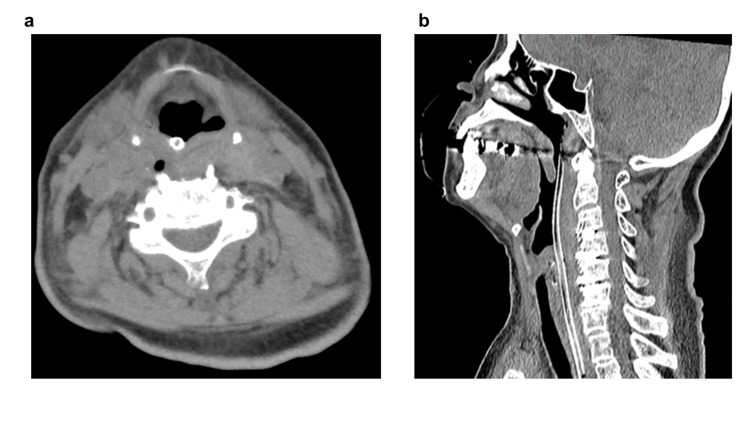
Follow-up computed tomography (CT) two days after surgery demonstrates complete resolution of the retropharyngeal hematoma with restoration of the airway. (A) Axial CT; (B) Sagittal CT
